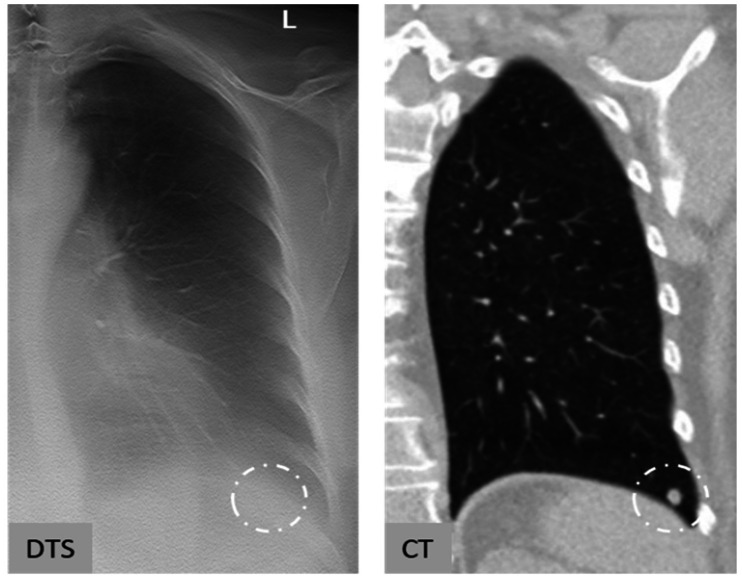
Example of a non-visible nodule on DTS. A 63-year-old man, no history of smoking but exposed to smoke at home, no history of cancer. Incidental, solid nodule with an average diameter of 8 mm in the left lower lobe. Volumetric estimate on CT was 128 mm^3^ on baseline and 208 mm^3^ on follow-up, with a volumetric change between the examinations of +63% and a VDT of 1815 days. The nodule was assessed as non-measurable by both observers on baseline and follow-up DTS. CT, computed tomography; DTS, digital tomosynthesis; VDT, volume doubling time.
